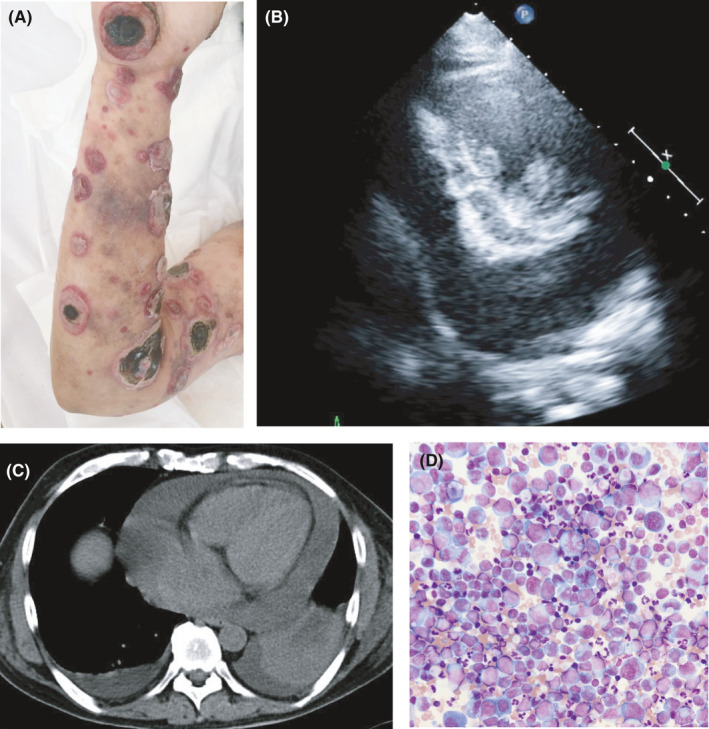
(A) Tumors due to MF were found in various parts of his extremities and trunk. The tumors were protuberant, 1–10 cm in size, and the aborted areas were necrotic. (B) Echocardiographic findings showed that the left ventricle was depressurized due to pericardial effusion. (C) Computed tomography findings showed pericardial and bilateral pleural effusions and pneumonia in the left lower lobe. (D) Cytology of the pericardial fluid showed malignant cells
